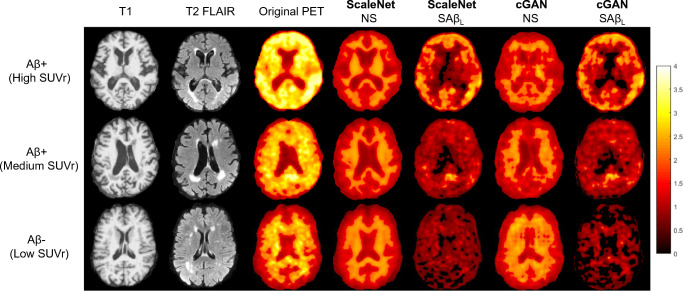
T1-weighted, T2-weighted FLAIR, and original PET images of patients visually classified as Aβ+, Aβ +, and Aβ− with high (top), medium (middle), and low (bottom) SUVr values. The NS images estimated from ScaleNet and cGAN, as well as derived SAβ_L_ volumes, are also shown. The PET and estimated images were set to the same image intensity range of 0–4 in hot-metal color-scale
